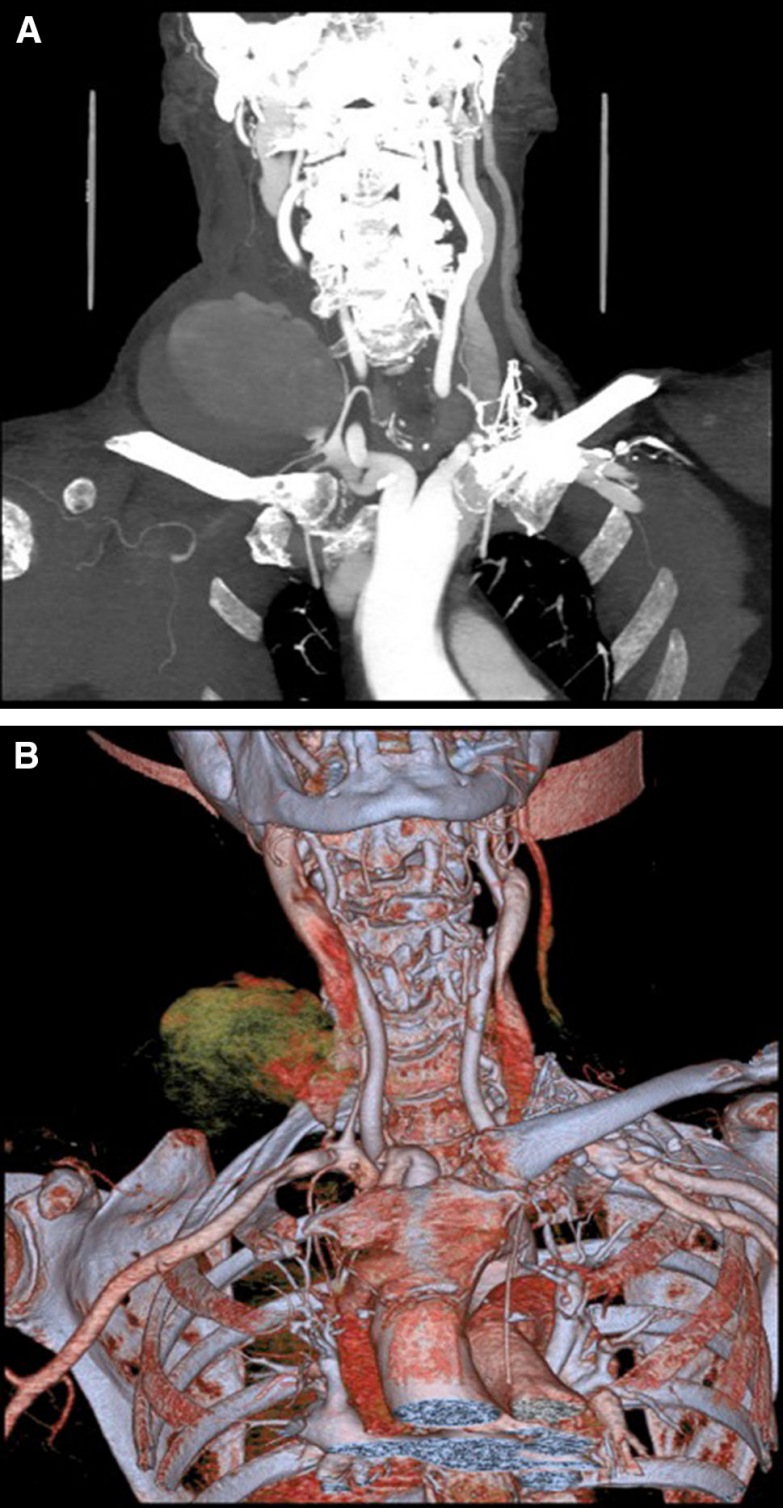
Coronal CT (A) and 2D reconstruction (B) of the upper chest and neck demonstrating large haematoma after inadvertent right subclavian artery puncture during right internal jugular vein line placement
